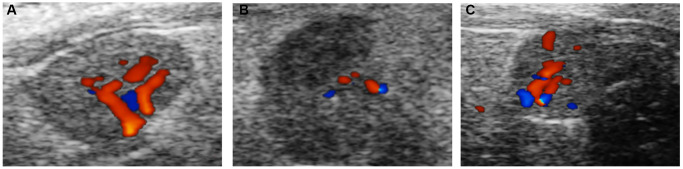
Ovarian blood flow under ultrasound. (A) Normal group; (B) Natural aging non-intervention group; (C) Natural aging intervention group.
